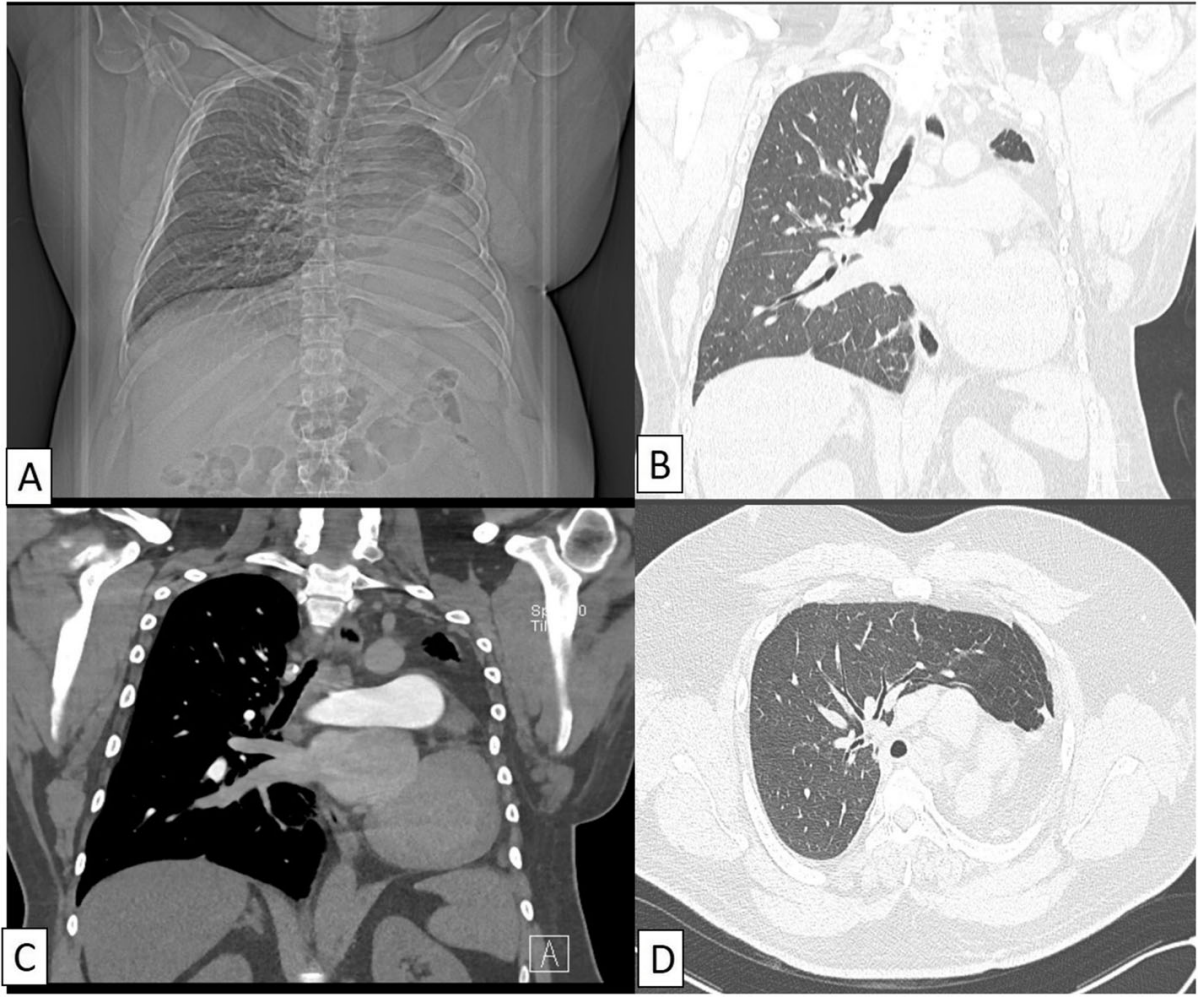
CT topogram (A) showing homogenous opacity of left hemithorax with non-bifurcation of trachea with mediastinal shift and contralateral herniation of right lung. CT images in coronal lung window (B), coronal mediastinal window (C), and axial lung window settings (D) showing absent left lung with shift of mediastinal structures into left hemithorax
